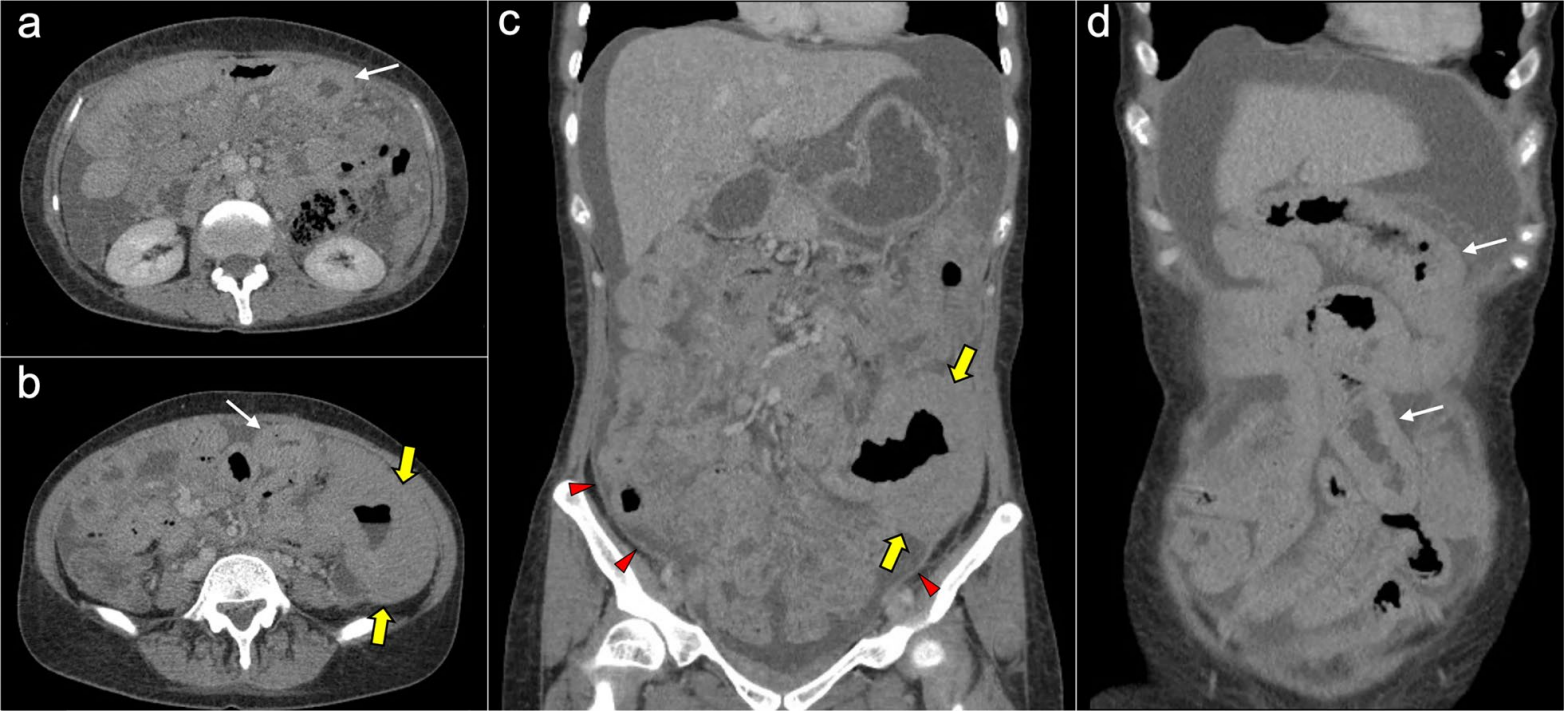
Peritoneal lymphomatosis in a 37-year-old woman with abdominal distension and loss of weight. Axial (a, b) and coronal (c, d) CT images in the portal phase show ascites, stranding of the mesenteric fat, diffuse thickening of the peritoneal folds (red arrowheads) and wall thickening of small bowel loops (white arrows). Note the presence of a mildly enhancing bulky mass in the left paracolic gutter with segmental encasement of the ascending colon (yellow arrows). The final diagnosis of B-cell non-Hodgkin's lymphoma was confirmed after ultrasound-guided biopsy of the bulky mass and cytology of the ascitic fluid
